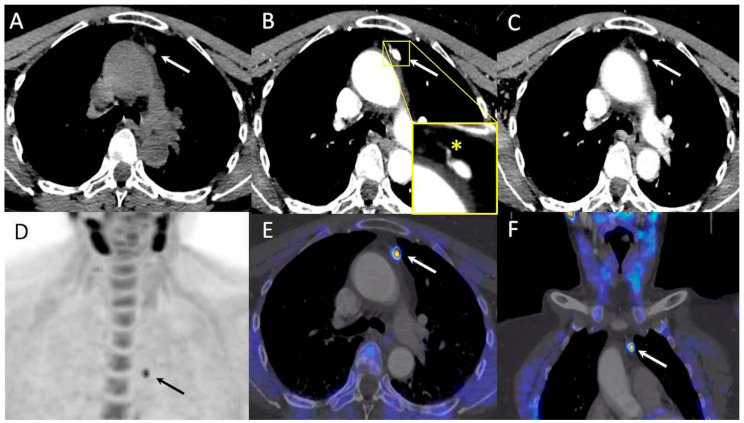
71-year-old woman with primary hyperparathyroidism (pHPT) and symptomatic osteoporosis. Preoperative positron emission tomography/four-dimensional computed tomography (PET/4D-CT) performed after inconclusive neck ultrasonography, identified a mediastinal nodule with high ^18^F-FCH uptake in front of ascending aorta (arrow), with typical contrast media enhancement profile. Hyperplasic parathyroid (0.3 g, 9 mm) was confirmed by pathological analysis. (A–C): four-dimensional computed tomography (4D-CT), axial slice: no-contrast-media computed tomography (CT), 45 HU; 45 sec post injection, 364 HU (yellow box: polar vessel sign); 70 sec post injection, 180 HU. (D): ^18^F-Fluorocholine positron emission tomography (^18^F-FCH PET), anterior maximum intensity projection (MIP); (E,F): PET/4D-CT, axial and coronal slice.
